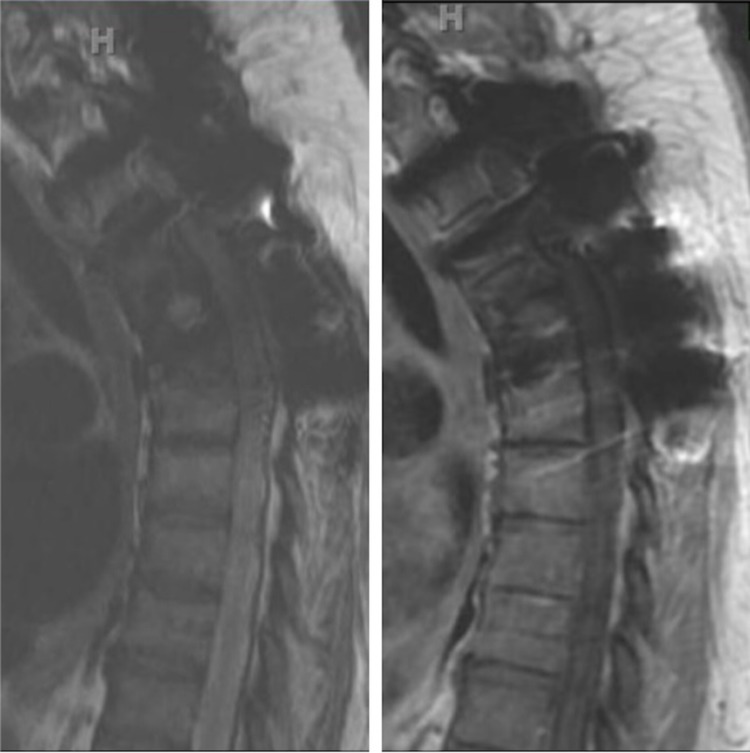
18-month postoperative T2 sagittal MRI (left) and T1 sagittal MRI with contrast (right) MRI (Magnetic Resonance Imaging)
